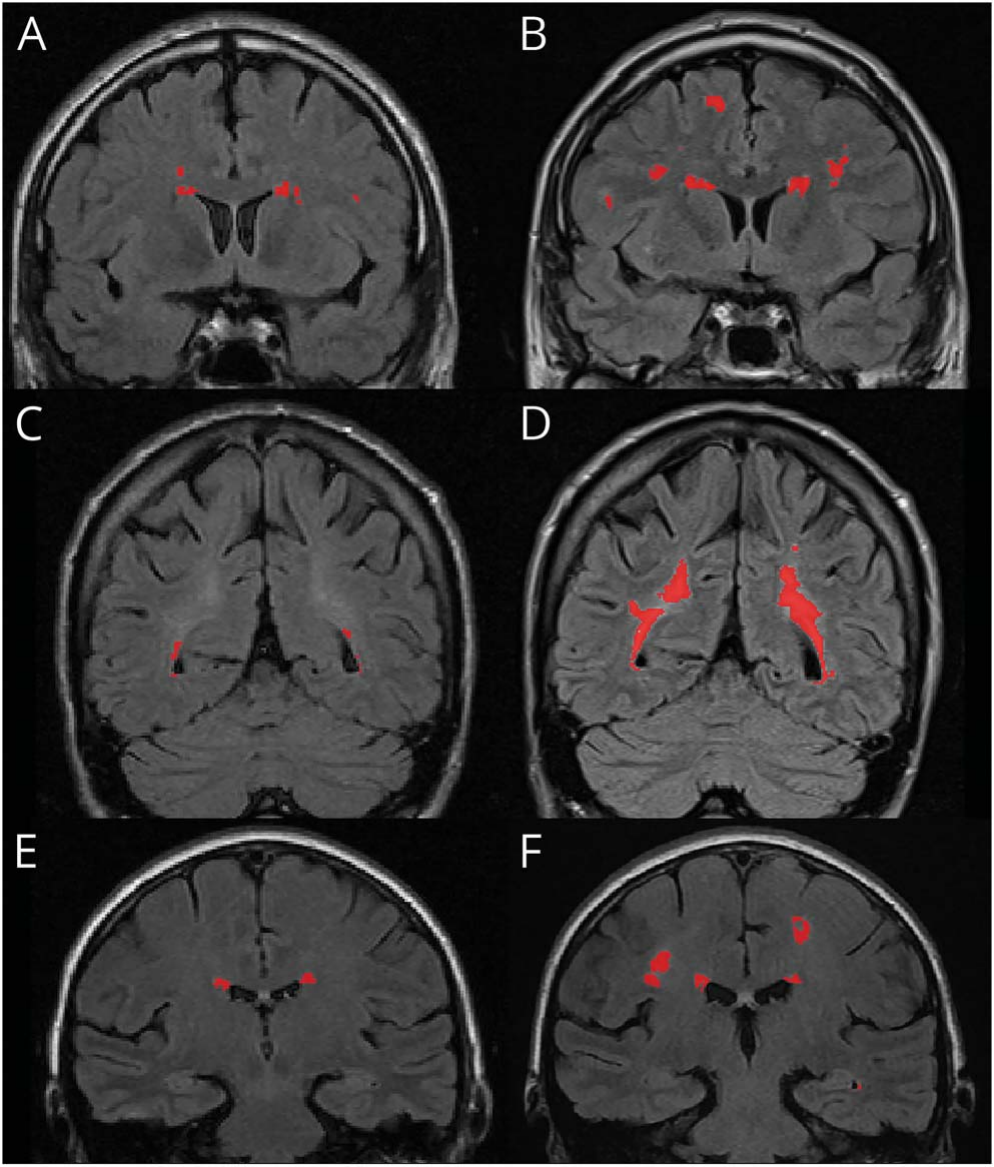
WMH progression in 3 patients with Fabry disease Coronal T2-weighted FLAIR MRIs with WMH highlighted in red by uom_overlay_editor software. MRIs were selected to demonstrate the WMH lesion mask created by uom_overlay_editor in patients with WMH progression. (A and B) FLAIR MRIs from the same participant taken after a 6-year follow-up period. (C and D) FLAIR MRIs from a second participant taken after an 8-year follow-up period. (E and F) FLAIR MRIs from a third participant taken after a 6-year follow-up period. FLAIR = fluid-attenuated inversion recovery; WMH = white matter hyperintensity.
